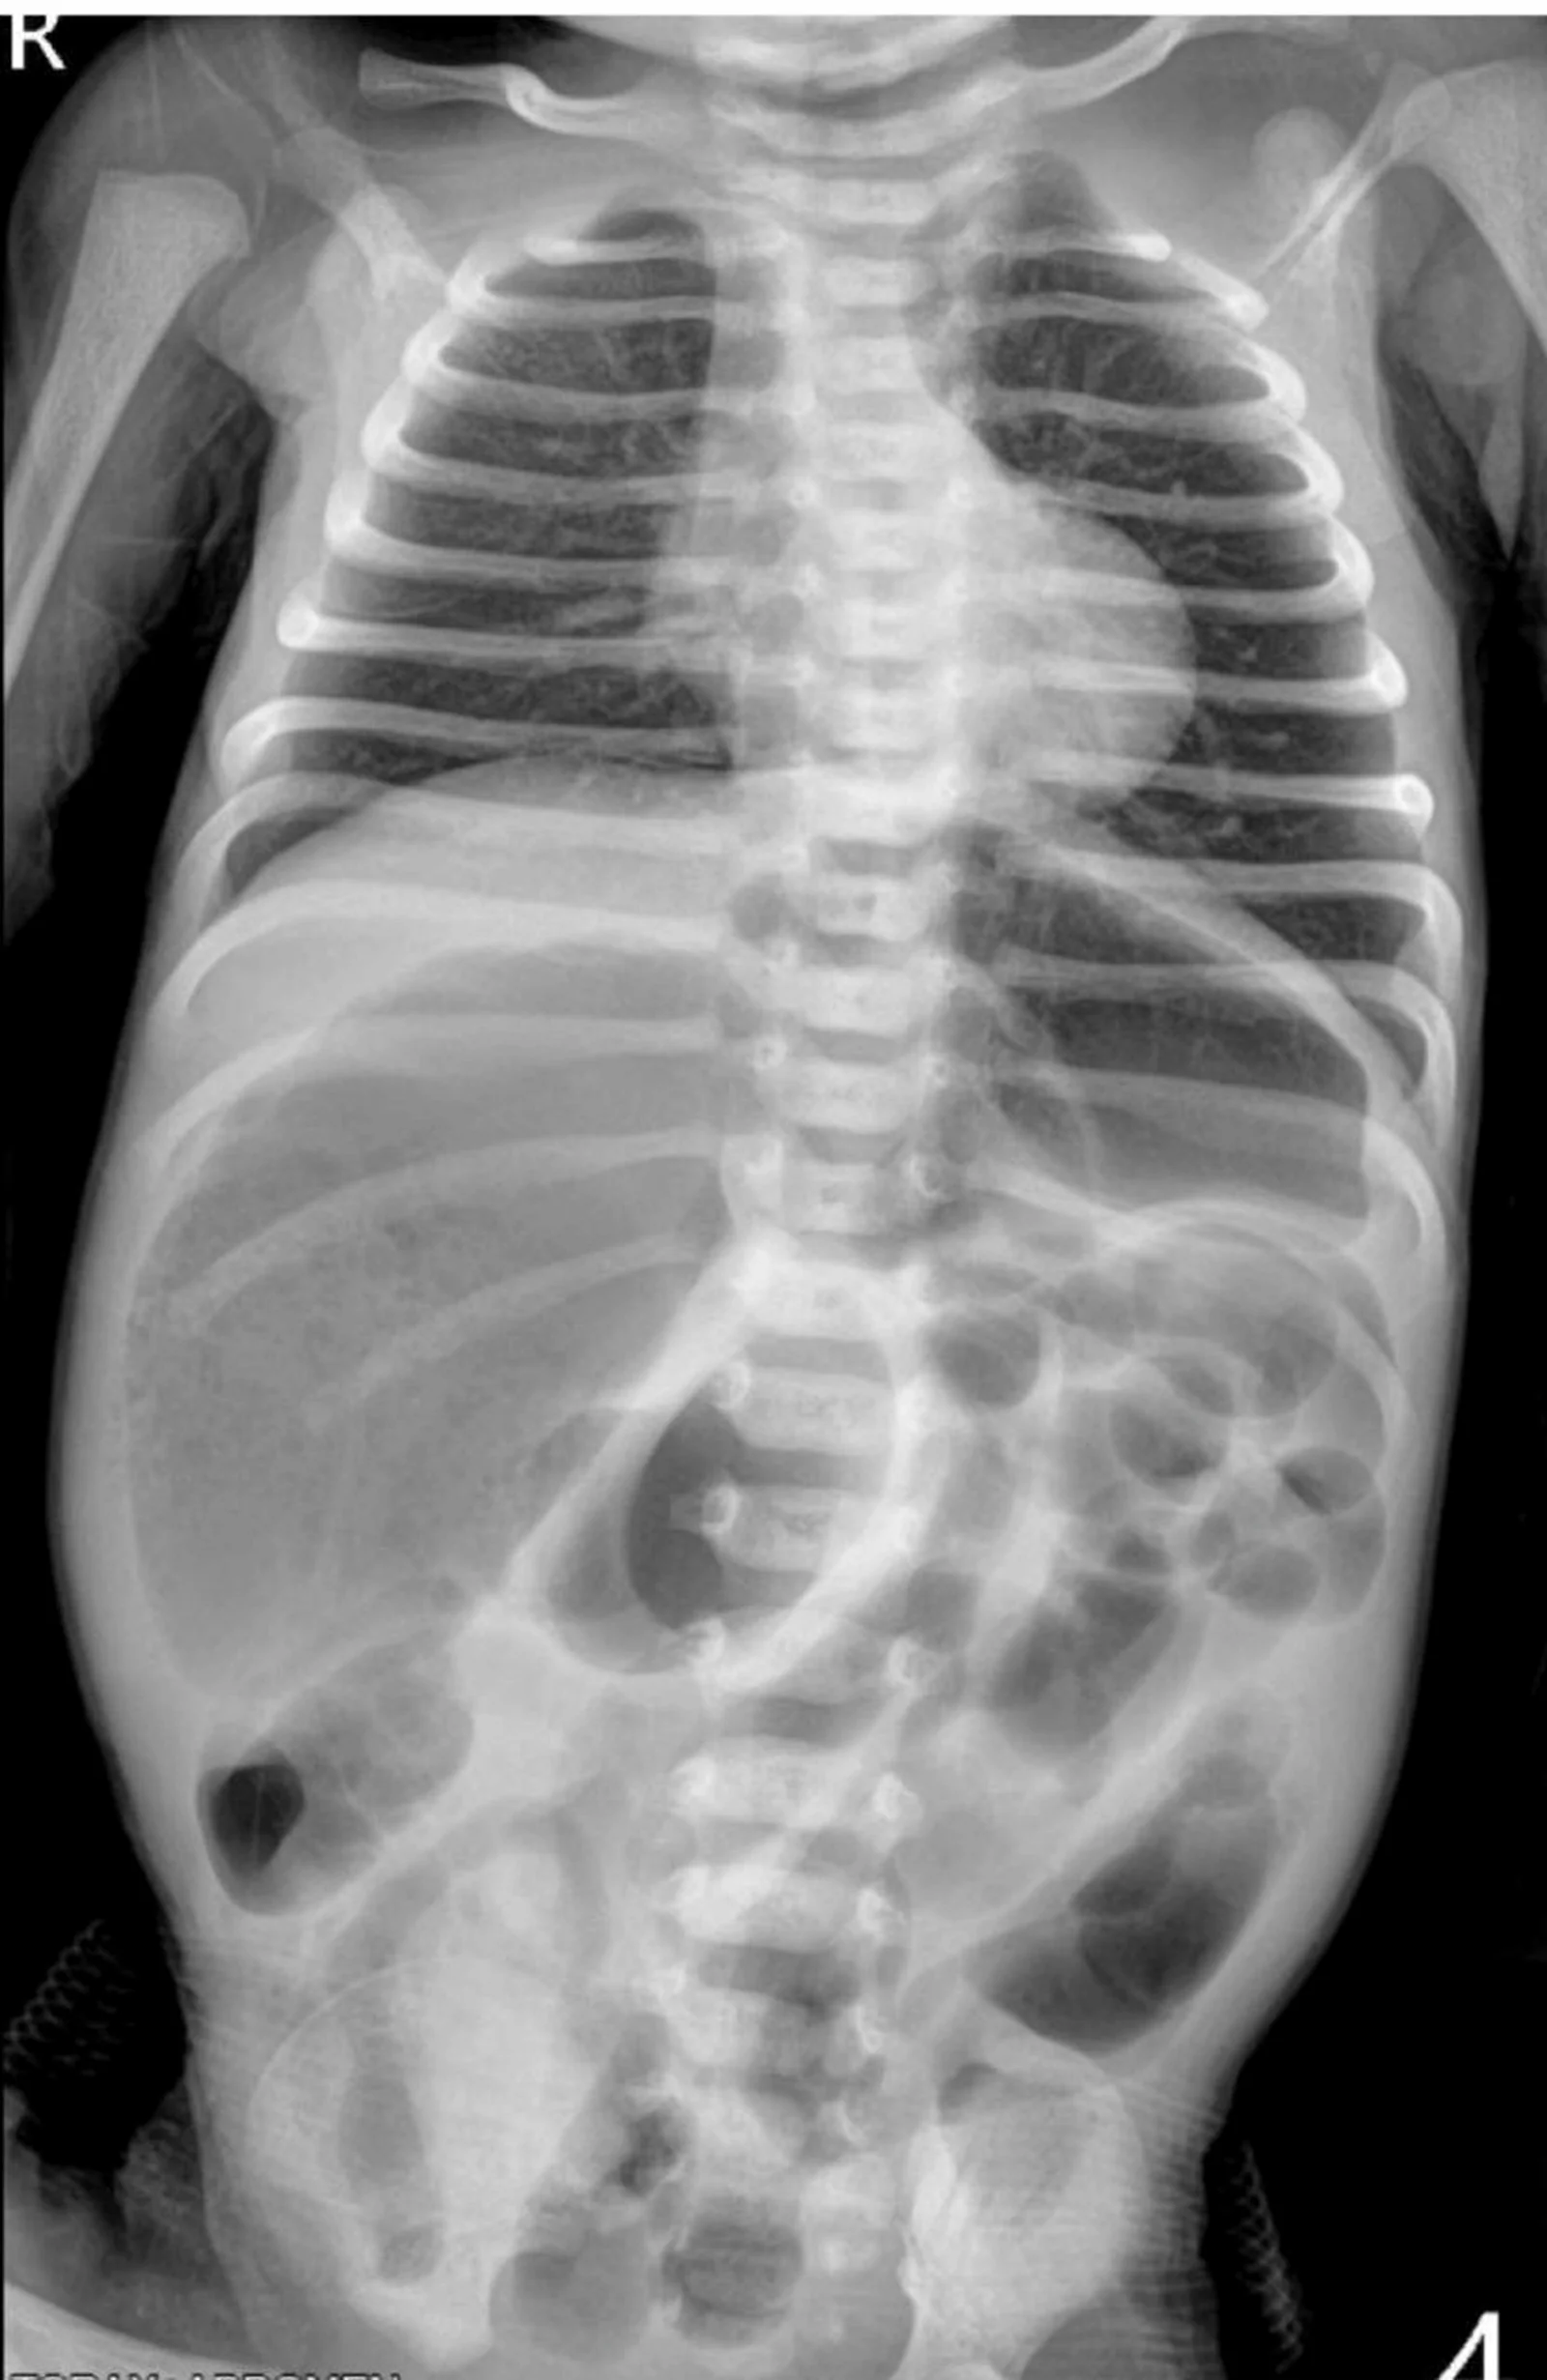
Abdominal radiograph Supine anteroposterior abdominal radiograph showing diffuse bowel-loop dilatation and relative paucity of distal gas, without evidence of pneumoperitoneum.
